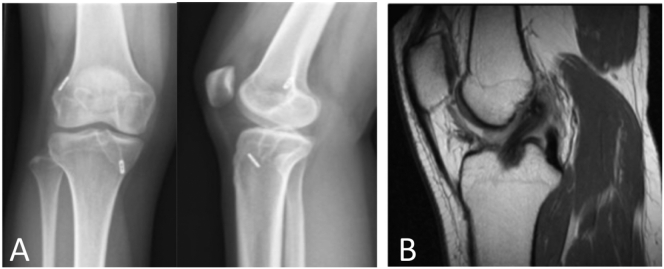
A. Plain X-rays AP and lateral right knee (patient 4). Adequate tunnel positioning. B. Sagittal MRI image of an intact ACL graft (patient 4).
